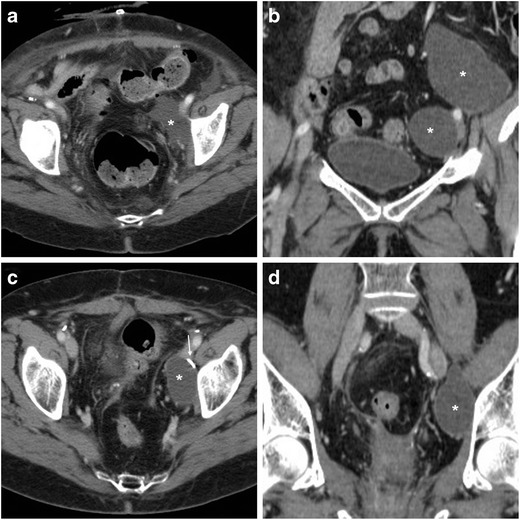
Usual CT appearance and short-time evolution of pelvic lymphoceles. A, B) Three days after AH for uterine body carcinoma, early CT (A) showed a fluid attenuation collection (*) without perceptible wall abutting the left external iliac vessels. Four weeks later, repeated CT (B) showed large left-sided lymphoceles (*) with unchanged homogeneous attenuation and development of a thin, regular wall. C, D) in another patient, early postoperative CT after radical AH showed a well-demarcated ovoid collection (*) with thin regular wall measuring 4 × 2.5 cm, adjacent to surgical clips (thin arrow in C) and left external iliac vessels
